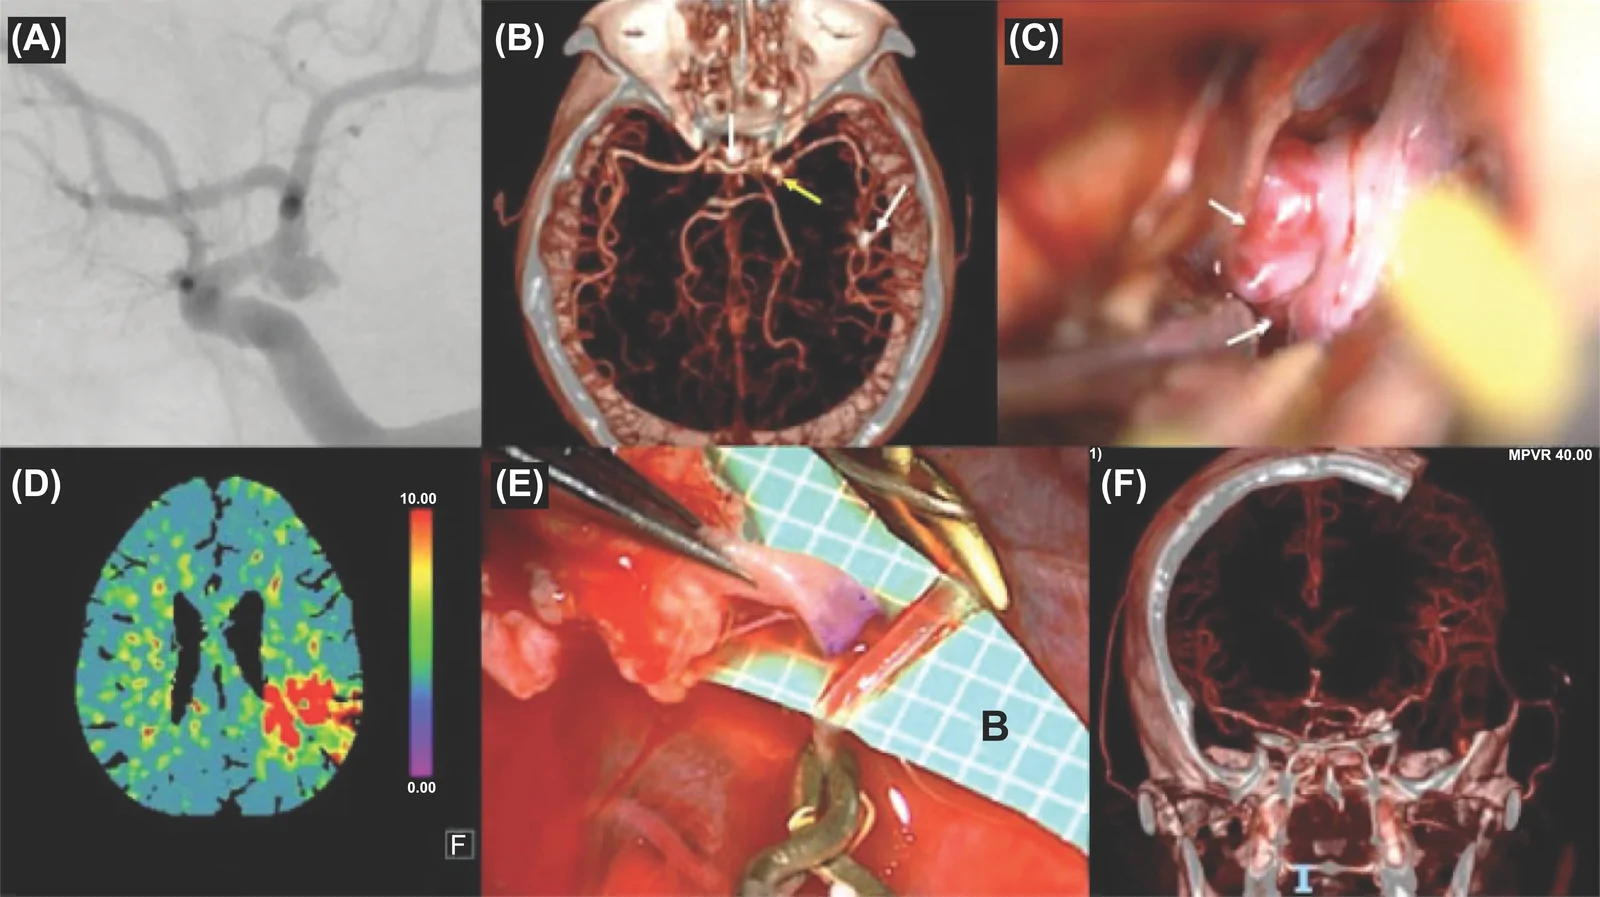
Case 1. (A) Oblique-view DSA reveals a bi-lobed configuration consistent with kissing aneurysms. (B) Preoperative CTA demonstrates migrated embolization coils (white arrows) and a persistent aneurysm sac (yellow arrow). (C) Intraoperative view confirms the presence of two separate aneurysms (arrows). (D) Perfusion CT shows prolonged transit time in the frontoparietal region related to the coil migration. (E) A low-flow superficial temporal artery–middle cerebral artery (STA–MCA) bypass was performed to support perfusion. (F) Postoperative CTA reveals restored MCA circulation; the decompressive craniectomy is evident.
